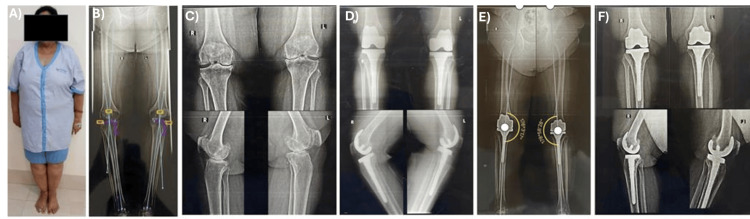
The post-operative orthoscanogram. (A) Morbidly obese patient undergoing CR RA-TKA with BMI 45; (B) pre-operative orthoscanogram with 12° varus deformity in both knees; (C) pre-operative radiographs - standing anteroposterior and lateral view; and (D) immediate post-operative standard anteroposterior and lateral view; (E) post-operative orthoscanogram showing hip-knee-ankle angle (right-4° varus and left-2° varus); and (F) post-operative follow-up radiograph at six months.
